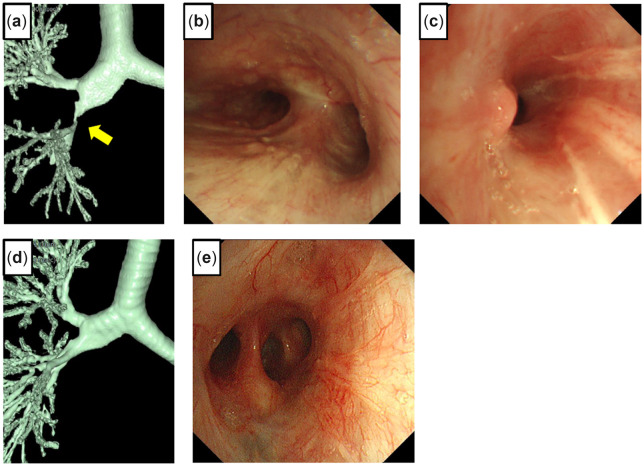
Three-dimensional computed tomography 6 months after a lung transplant shows severe stricture of the truncus intermedius (TIM) (a). Bronchoscopy 6 months after the lung transplant shows healing with complete lumen patency of the right main bronchial anastomosis (b) and severe stricture at the distal side of the TIM (c). A 3-dimensional computed tomography scan 2 years after TIM reconstruction shows lumen patency (d). Bronchoscopy 2 years after reconstruction shows healing of the anastomosis (e).
